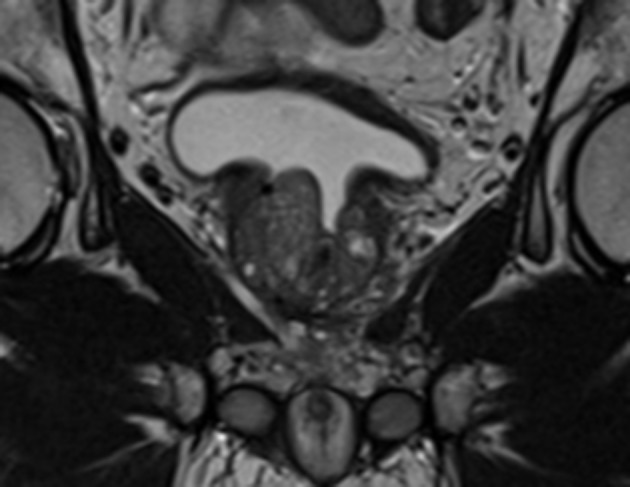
Coronal T2-weighted MRI revealing visible TURP defect.
